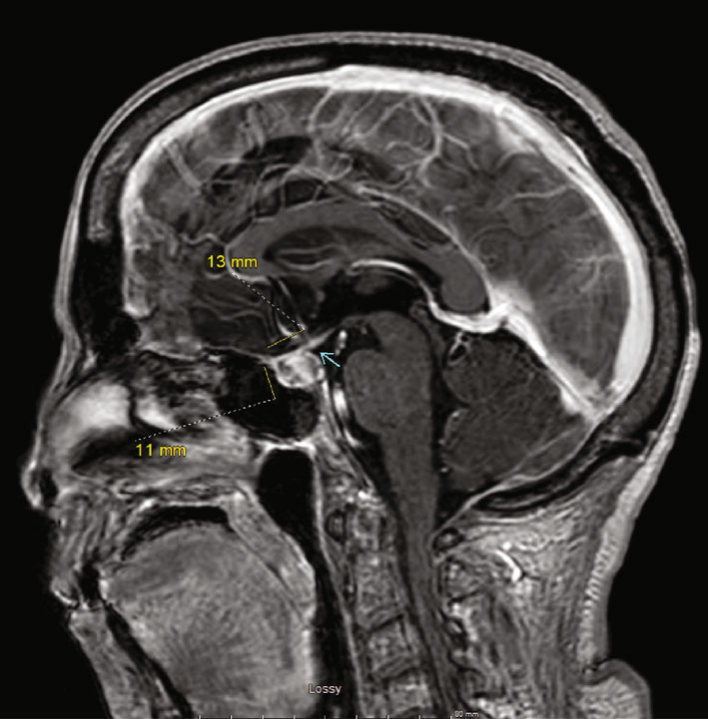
MRI done during the hospital admission. The blue arrow points to changes in the pituitary gland.
